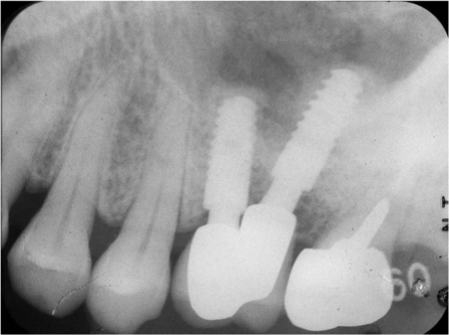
Case 1. Periapical X-ray film, April 2014. Seven years and 5 months after superstructure placement, showing no bone resorption around the double implant necks
